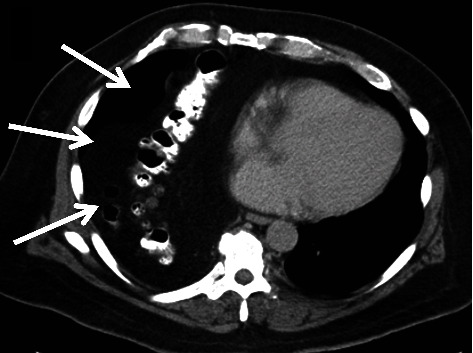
CT scan: colon and omentum lodged within the right chest (white arrows).
